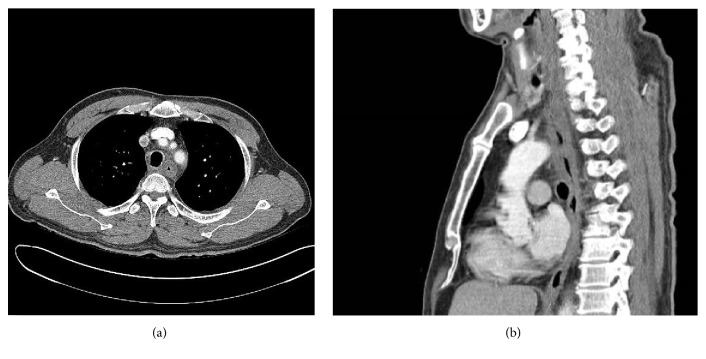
Chest computed tomography (CT). Transverse plain (a) and sagittal plain (b) images of the chest CT showed diffuse esophageal wall edema and thickening. However, esophageal perforation or mediastinitis was not detected.
